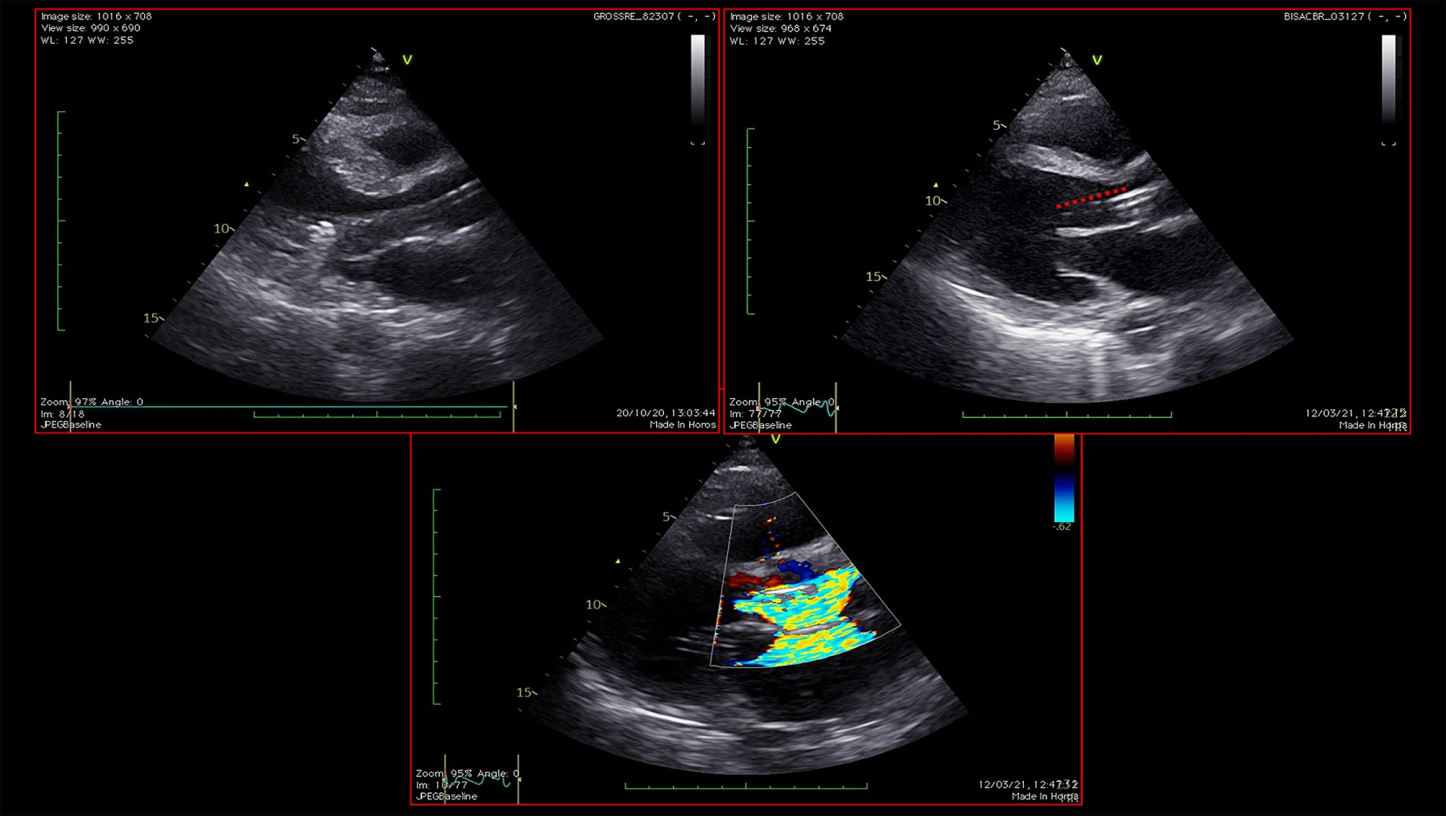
Echocardiographic assessment during mechanical circulatory support with Impella. This picture shows the echocardiographic assessment of cannula position during Impella support in two different patients. The distance from the inlet of the cannula and the aortic root, which should be around 35 mm in parasternal long axis view (top pictures), should be routinely measured in order to monitor cannula position and assess possible cannula dislocation. The picture at the bottom shows the outlet of the motor in the ascending aorta, visualized with the aid of Color Doppler
